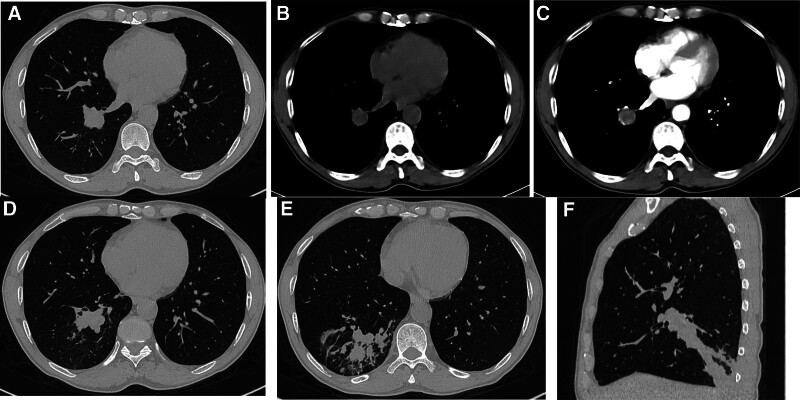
Images of on January 6, 2020 enhanced-chest CT, demonstrating mass shadow was observed in the basal segment of the lower lobe of the right lung (A, B), uneven enhancement on enhanced scanning (C), local bronchial stenosis and occlusion, multiple solid shadows, patches and cable shadows in the distal lung field (D–F). CT = computed tomography.
